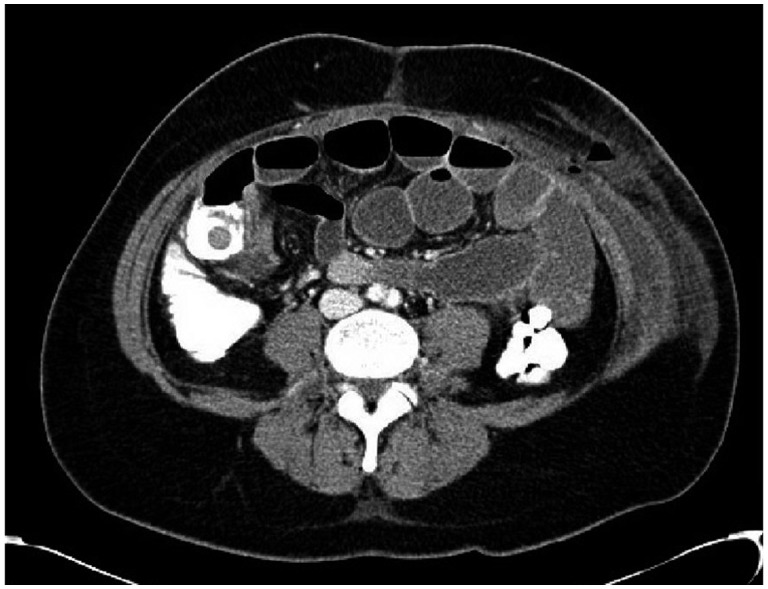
Contrast CT, transverse view of abdomen.
